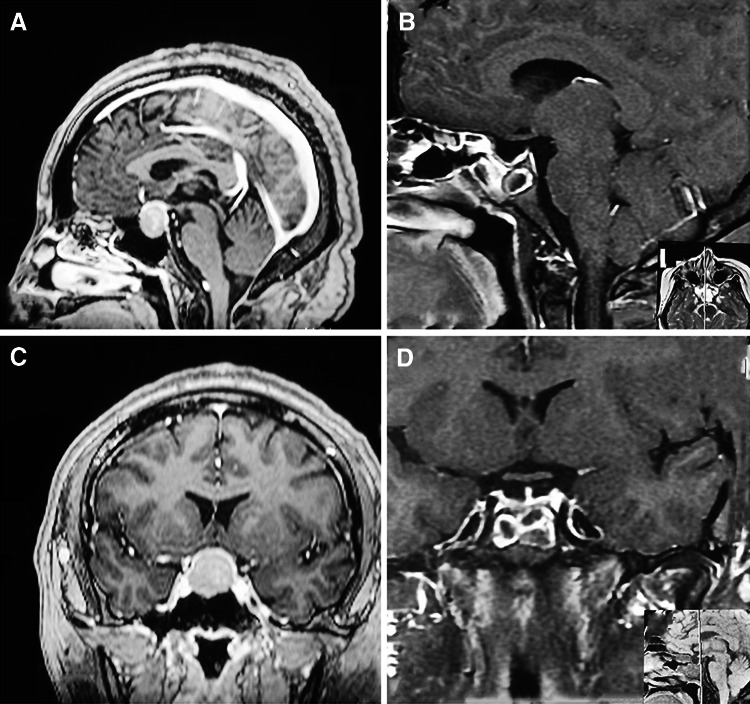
MRI images of one ACTH adenoma. A, C Before surgery, B, D gross total resection after surgery
